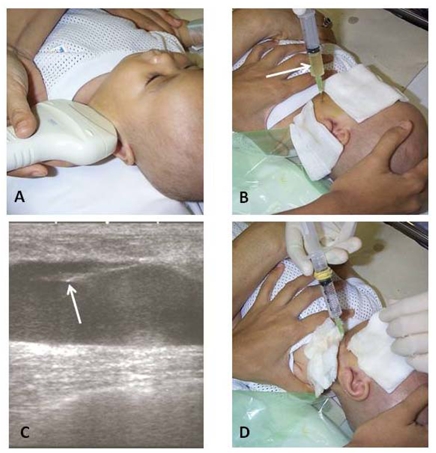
Intralesional bleomycin therapy. (A) Ultrasound assessment before injection. (B) Aspiration of cyst content reveals clear serous fluid (arrow). (C) Ultrasound image showing the tip of aspirating needle (arrow) within the cyst, and (D), while keeping the tip of the needle within the cyst, bleomycin aqueous solution is administered.
